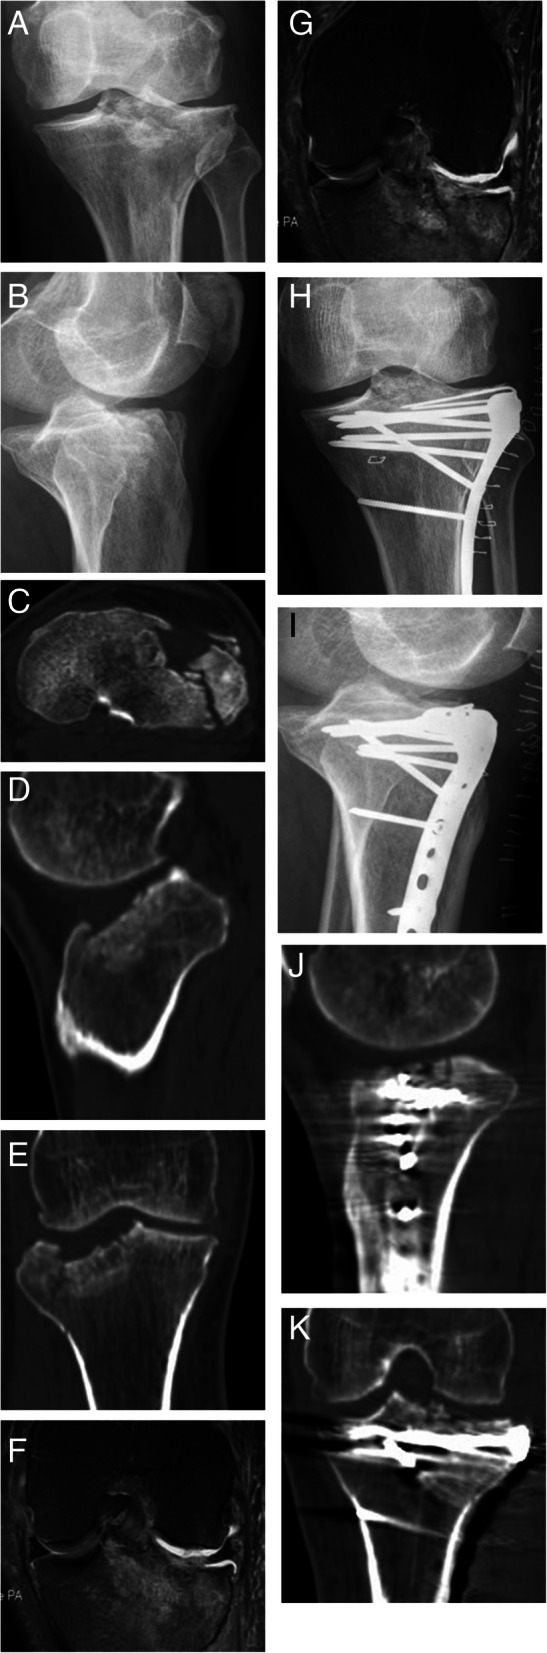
F, 56Y, anterior, and lateral tibial plateau fractures with MCL injury. a, b Preoperative x-rays of the tibial anterolateral plateau fracture.c–e. Preoperative CT shows anterolateral tibial plateau collapse and splitting, significant change in pTSA. f, g Preoperative MRI shows medial collateral ligament damage. h–k Postoperative x-ray and CT show an anatomical reduction of tibial plateau fracture
